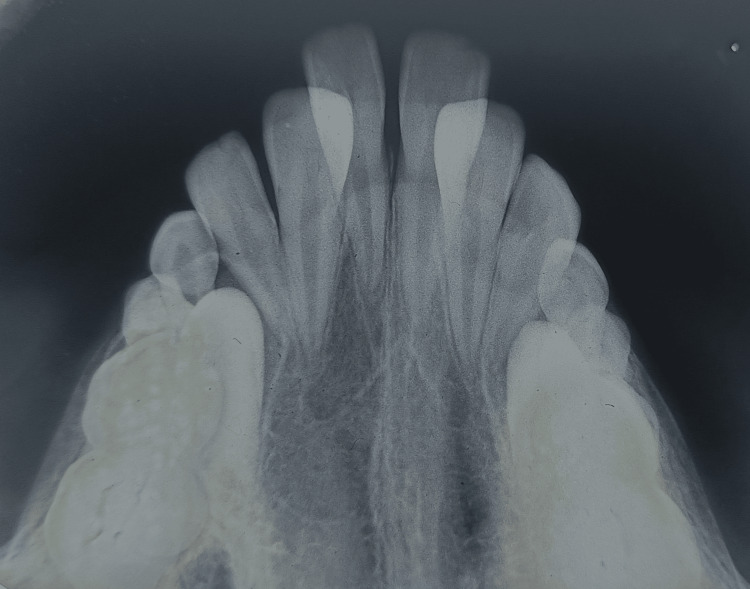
Pretreatment occlusal radiograph
